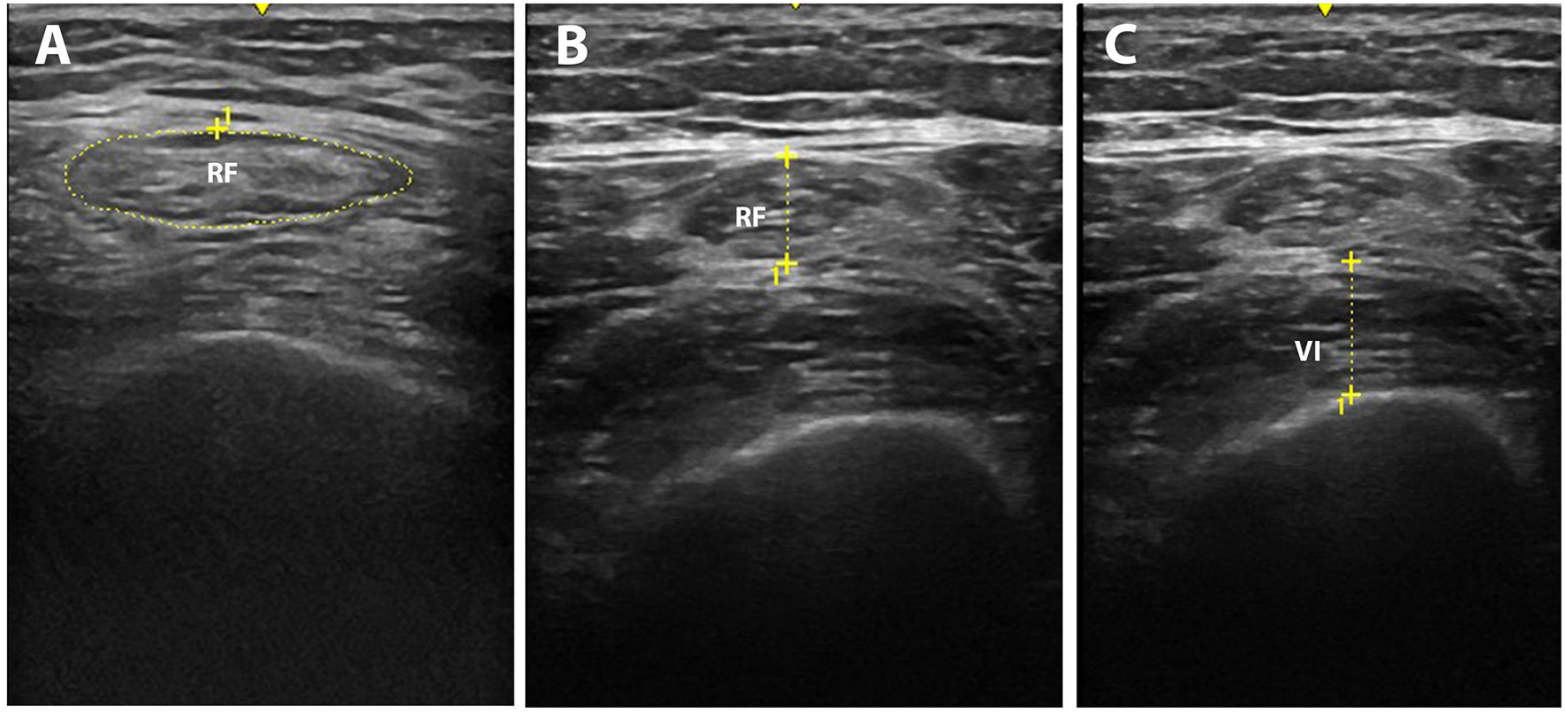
Representative muscle ultrasound image methods: rectus femoris cross-section area (A), the thickness of the anterior compartment of the quadriceps muscle [rectus femoris (B) and vastus intermedius (C)].
